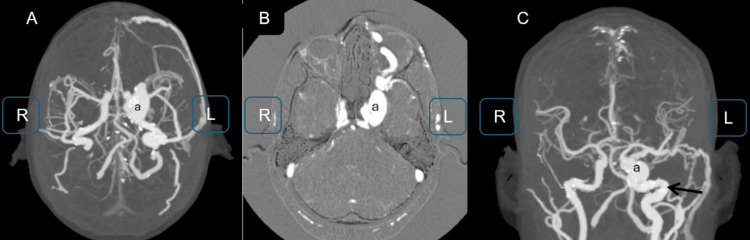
CTA with 3D and maximum intensity projection reconstructions: (A, B) axial images demonstrating a direct, high-flow CCF (a), characterized by early opacification and enlargement of the left CS in direct communication with the left ICA (arrow); (C) Coronal projection highlighting the fistulous point (a) and associated abnormal venous drainage, consistent with a Barrow type A fistula 3D: three-dimensional; CTA: computed tomography angiography; L: left; R: right; CCF: carotid-cavernous fistula; CS: cavernous sinus
